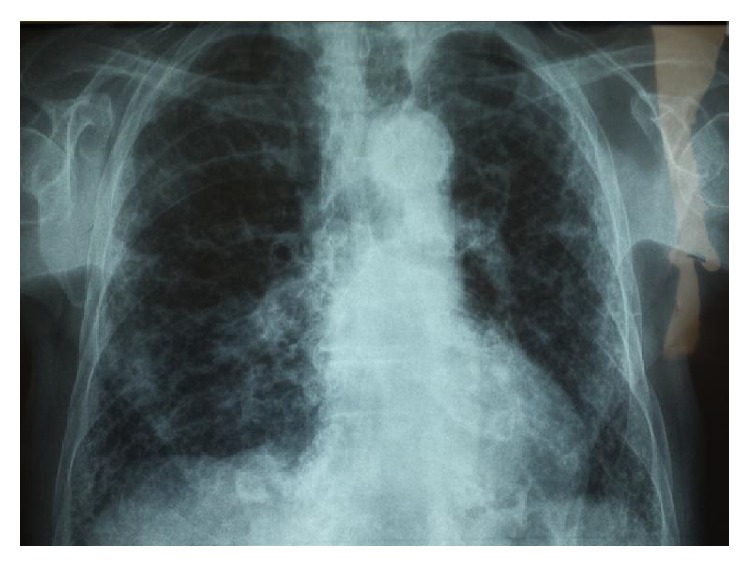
Current X-ray displaying trachea enlargement accompanied by fibrotic elements in the lungs.
